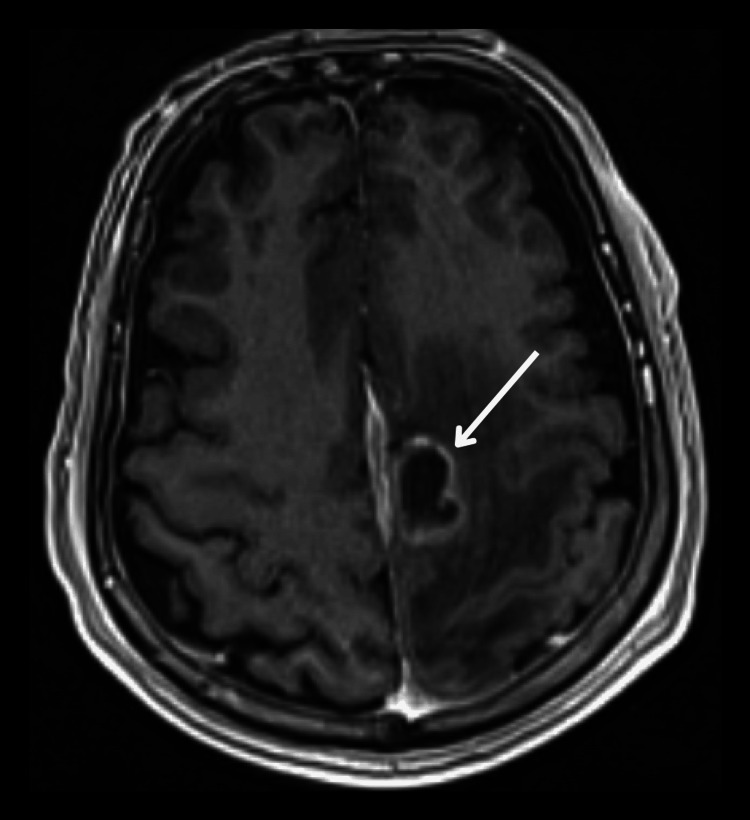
Initial presentation and early progression on osimertinib. Axial T1-weighted contrast-enhanced MRI brain image showing a solitary 2 cm enhancing lesion in the left frontal lobe at the time of disease progression on osimertinib. This lesion was surgically resected and found to harbor both EGFR exon 19 deletion and acquired EML4::ALK fusion. EGFR: epidermal growth factor receptor; ALK: anaplastic lymphoma kinase.
